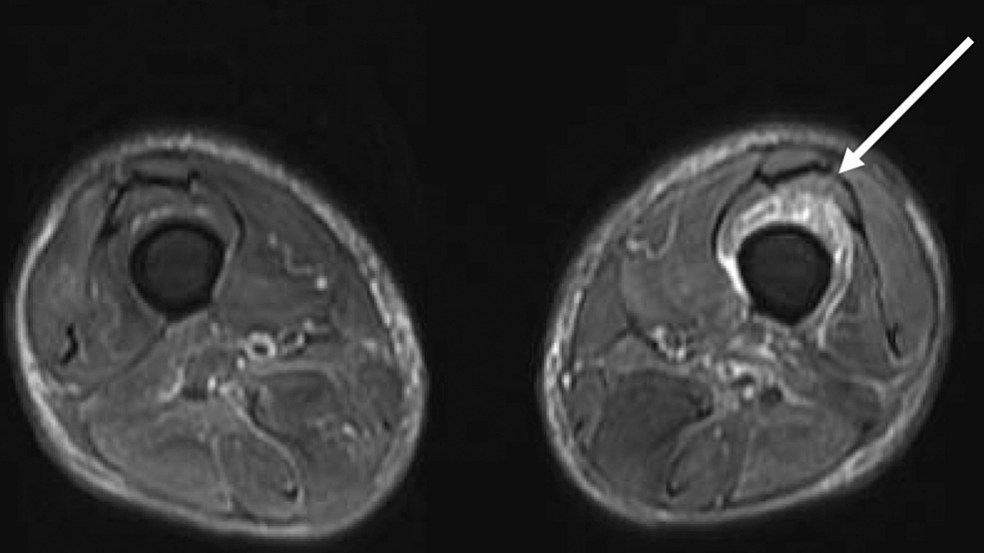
Magnetic resonance imaging (Short-TI Inversion Recovery) showing high signal in the vastus medialis muscle of the left (white arrow)
